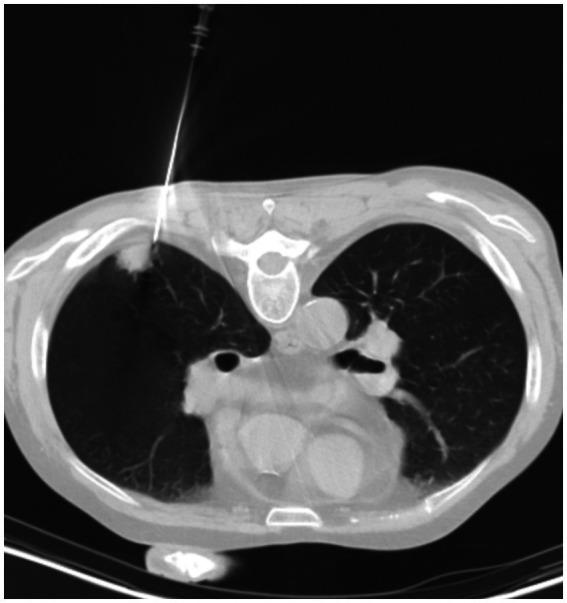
A 58-year-old male PC patient with a history of kidney transplantation 2 years ago. Chest CT in the lung window shows the process of percutaneous lung needle biopsy (PCNB).
